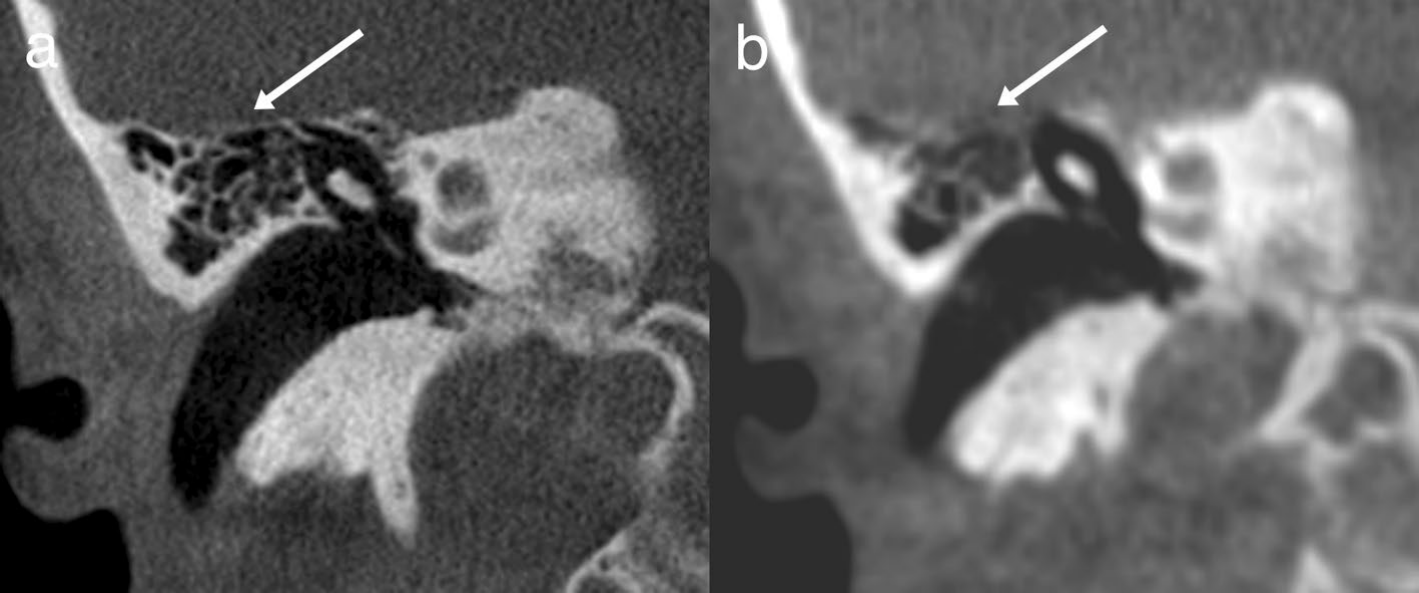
Example images in the coronal plane from a single patient, one taken with HR CBCT (a) and one with ULD CBCT (b). Fine bone structures, such as tegmen tympani, are not always identifiable in the ULD CBCT image
